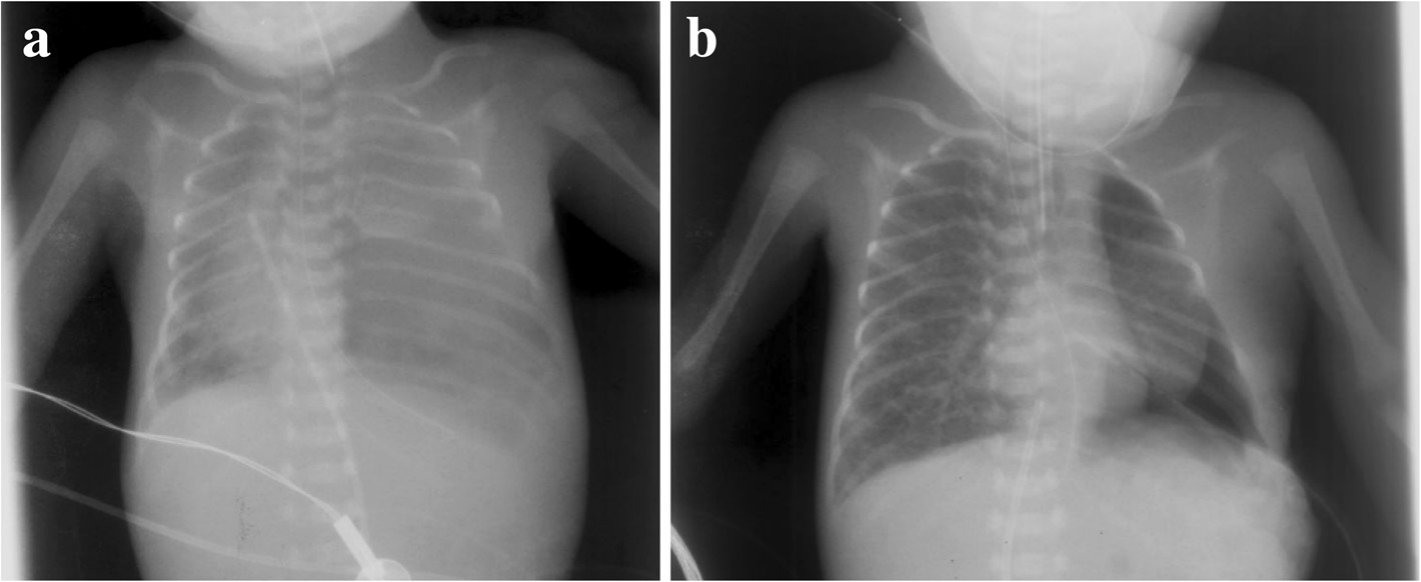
Preoperative (a) and postoperative (b) x-rays of the index patient
